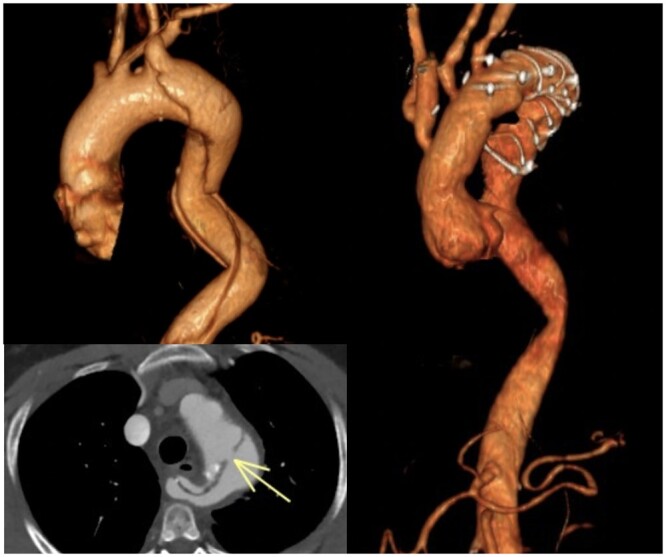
Computed tomography angiogram of a patient with type B acute aortic dissection treated with a Frozen Elephant Trunk.
